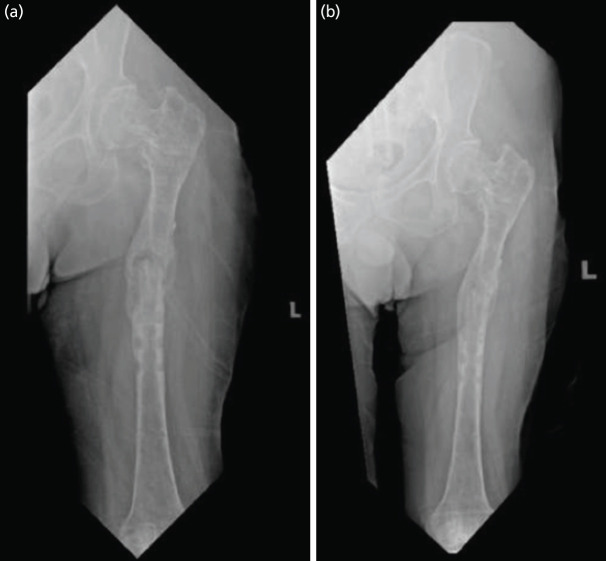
AP radiograph of Left femur showing (a) good union at fracture site after five months, (b) good consolidation at fracture site after eight months after femoral functional brace.
